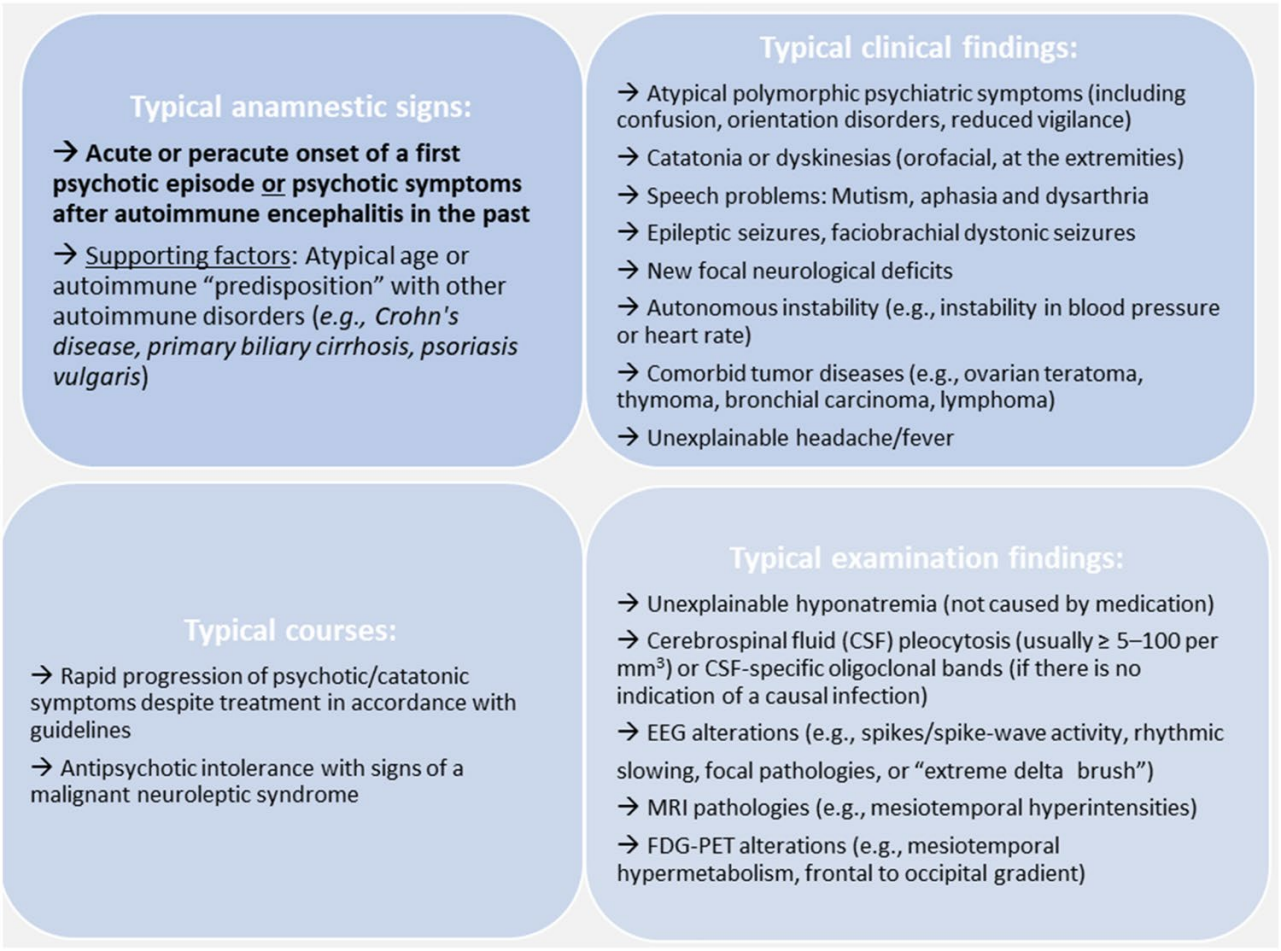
Red flags that should lead to antibody diagnostics (according to [2, 5, 22, 36, 53, 55, 61, 67, 74, 76, 77, 85]). *EEG* electroencephalography, *MRI* magnetic resonance imaging
